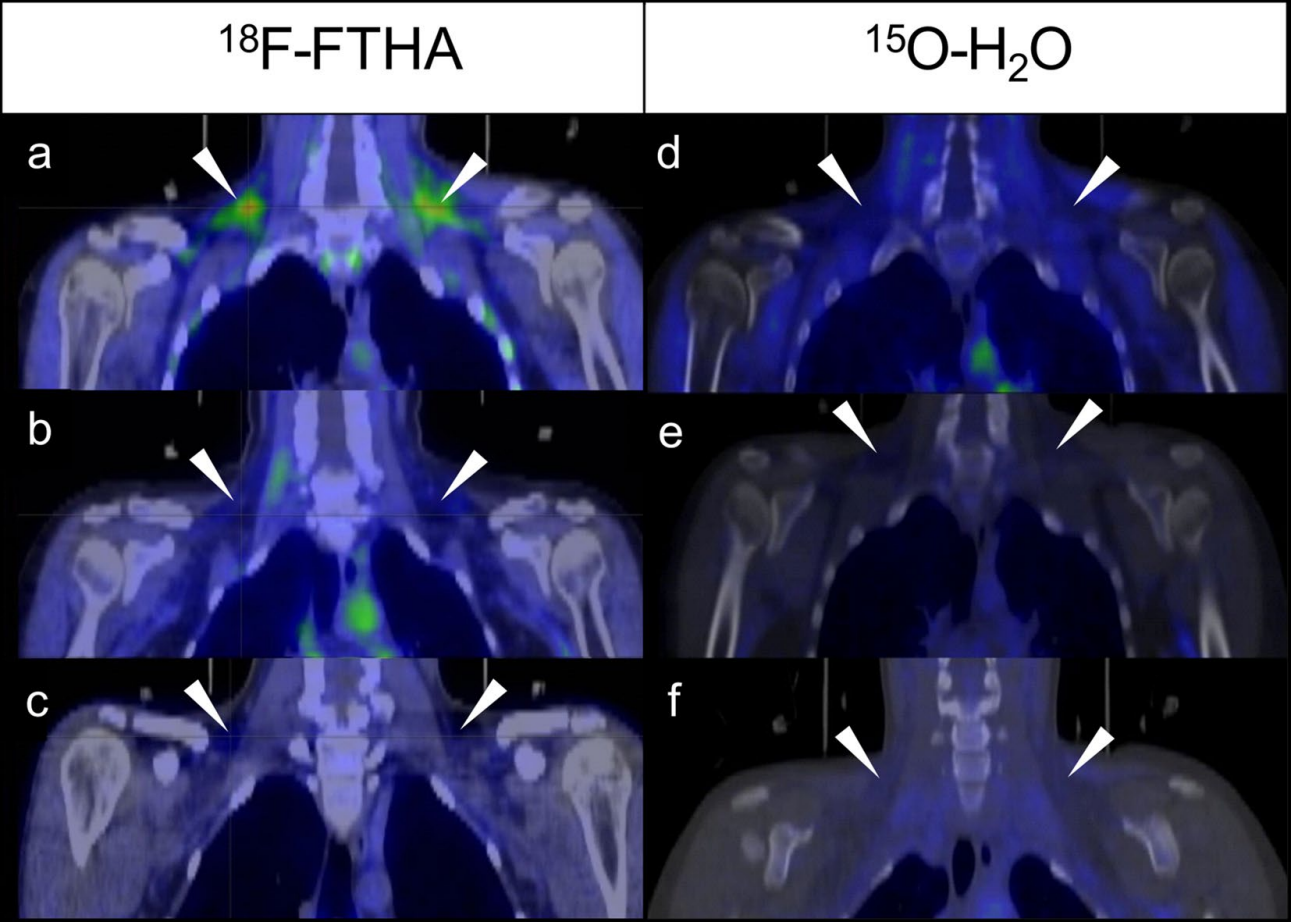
^18^F-FTHA (a–c) and ^15^O-H_2_O (d–f) PET/CT fusion images. Triangles indicate location of supraclavicular BAT depots. (a) BAT^(+)^ subject in cold exposure. Symmetrical activation of BAT can be seen in the supraclavicular adipose deposits. (b) BAT^(+)^ subject in room temperature. (c) Lean BAT^(−)^ subject in cold exposure. No activity in the supraclavicular region. (d) BAT^(+)^ subject in cold exposure, showing increased perfusion in supraclavicular area. (e) Same BAT^(+)^ subject as in image D in room temperature, minor supraclavicular activity. (f) BAT^(−)^ subject in cold exposure.
